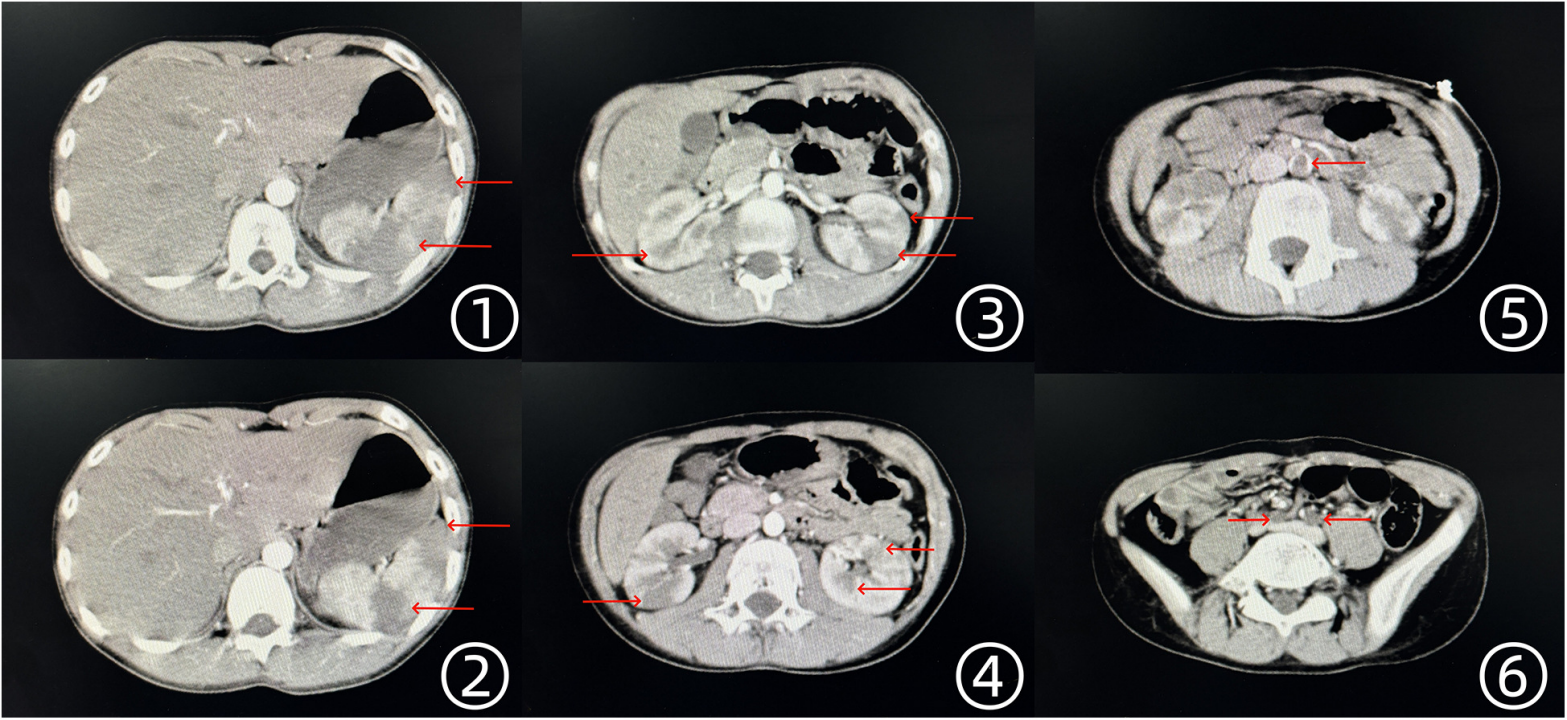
Enhanced CT scan findings of the thoracic and abdominal aorta. ①, ② Multiple low-density infarct foci in the spleen. ③, ④ Multiple low-density infarct foci in both kidneys. ⑤ Thrombus visible in the abdominal aorta. ⑥ Embolism in the bilateral iliac arteries, with absent visualization.
